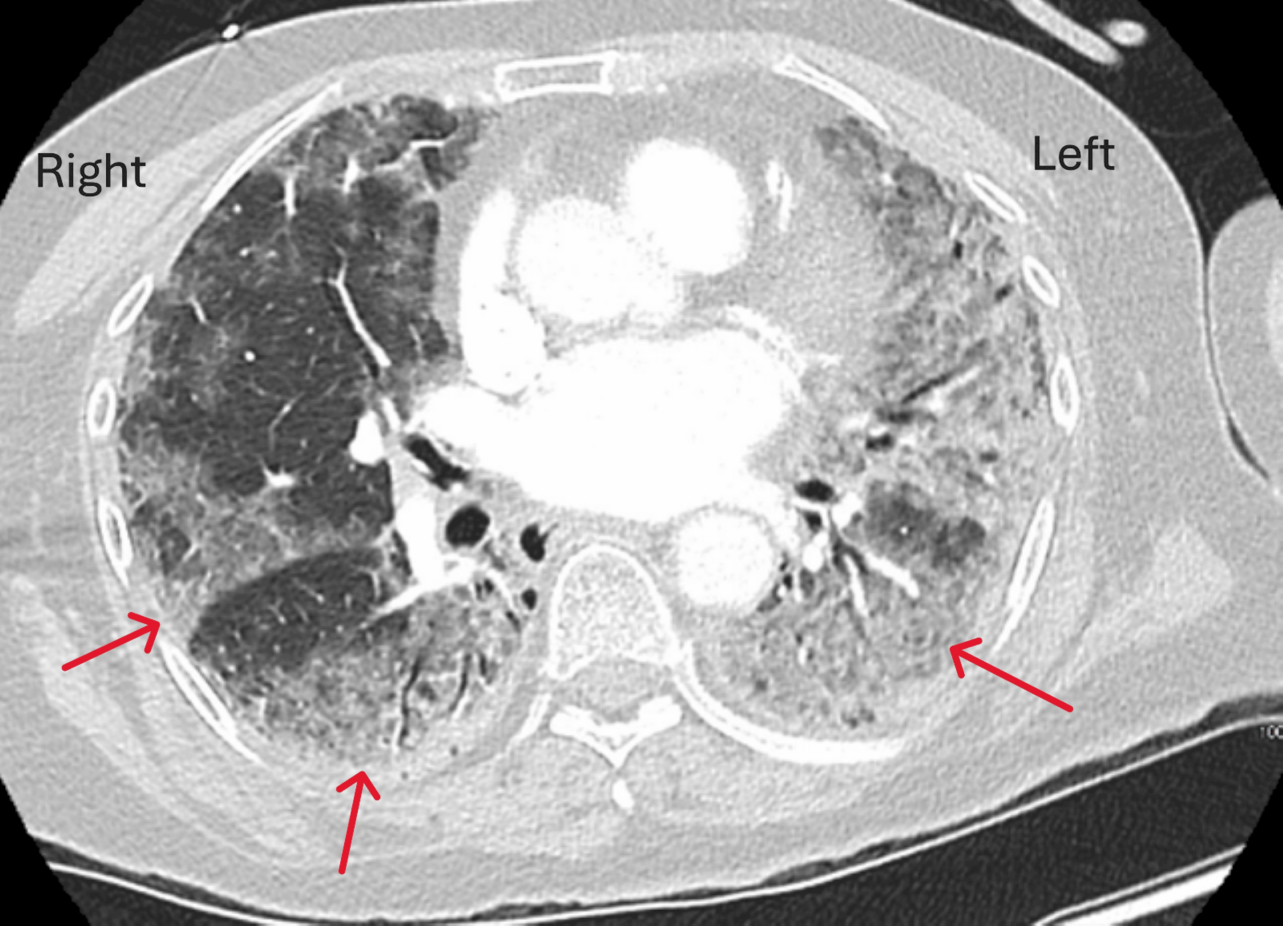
CT chest showing diffuse pulmonary infiltrates, with the entire left lung involved and patchy infiltrates in the right lung CT, computed tomography
